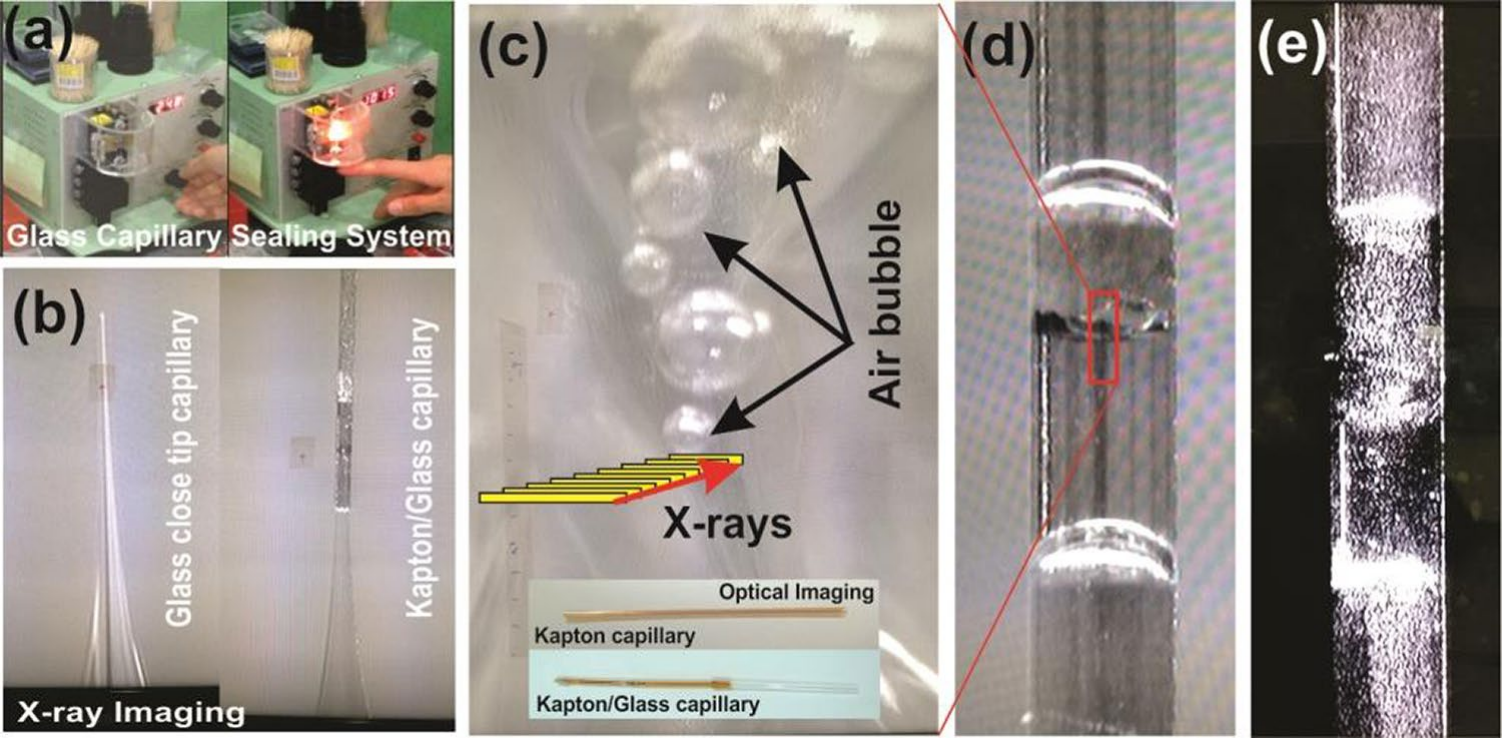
Glass capillary sealing system (a) showing: X-ray imaging of Kapton/glass capillary filled with precursor solution (b), Optical imaging showing the production of air bubbles during X-ray irradiation (c), images of Kapton capillary at beginning (d) and after the exposure (e) of 6.7 keV monochromatic X-rays under laser-light exposure.
